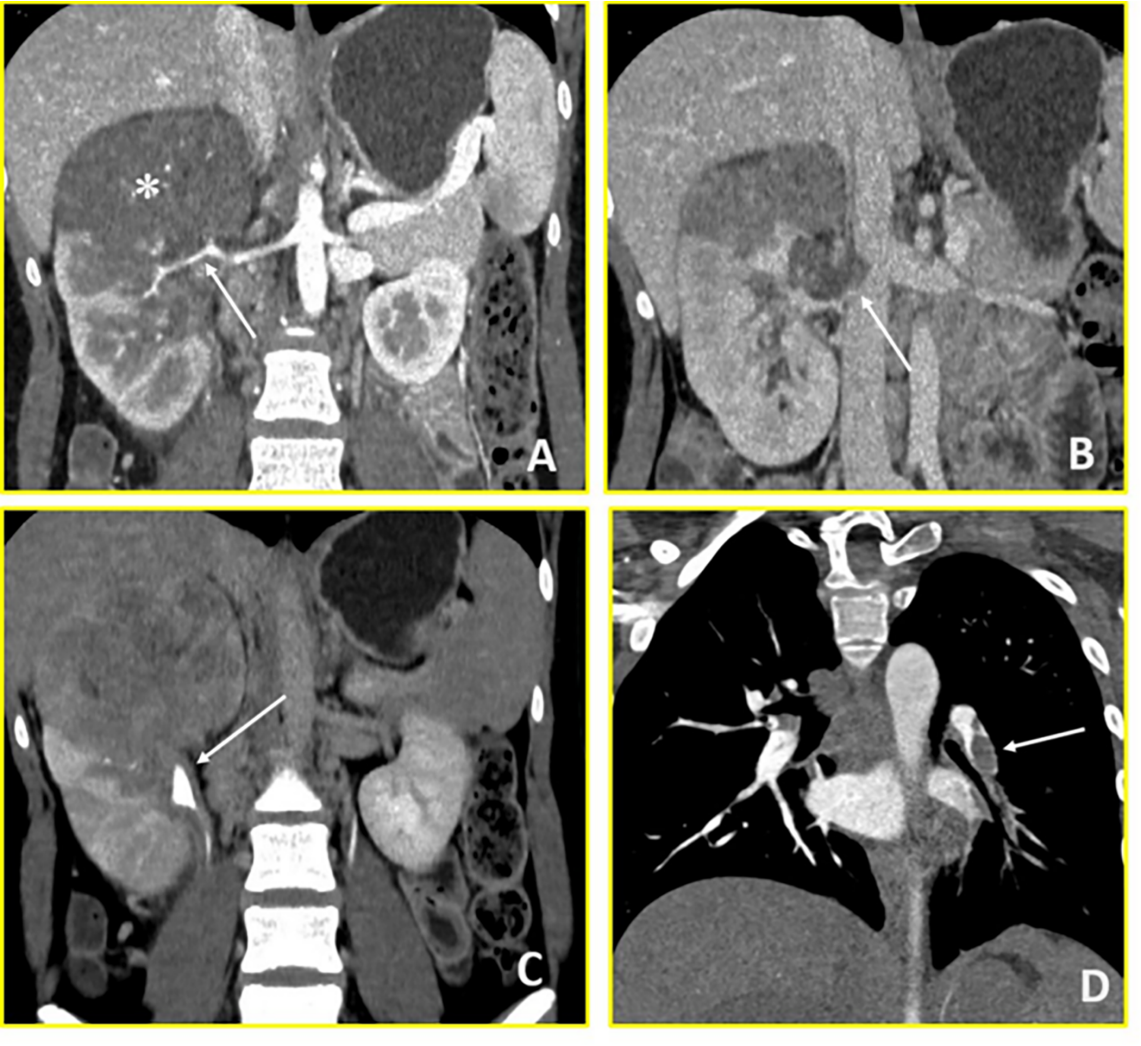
Wilms’ Tumour (Case 4). Contrast-enhanced CT imaging demonstrates a hypovascular right renal mass with vascular invasion and distant thrombotic complications. (A) Coronal arterial-phase image reveals a predominantly hypovascular mass in the right kidney (asterisk) with infiltration of the right renal artery (arrow). (B) Coronal venous-phase image shows tumor thrombus in the right renal vein with extension into the inferior vena cava (arrow). (C) Coronal urographic-phase image demonstrates infiltration of the renal collecting system with involvement of the calyces (arrow). (D) Coronal arterial-phase image of the chest reveals a filling defect in the left interlobar pulmonary artery (arrow), consistent with pulmonary thromboembolism.
